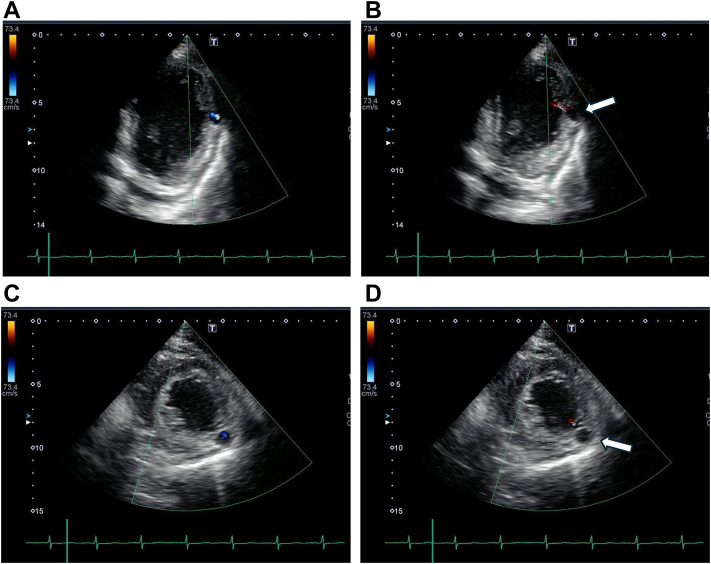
Echocardiography on Day 26 With Color Doppler Demonstrating Bidirectional Flow Communication (A) Long-axis view during systole. (B) Long-axis view during diastole. (C) Short-axis view during systole. (D) Short-axis view during diastole. Bidirectional flow through the narrow neck confirms communication between the left ventricle and pseudoaneurysm. Arrows in B and D indicate the pseudoaneurysm with flow communication.
